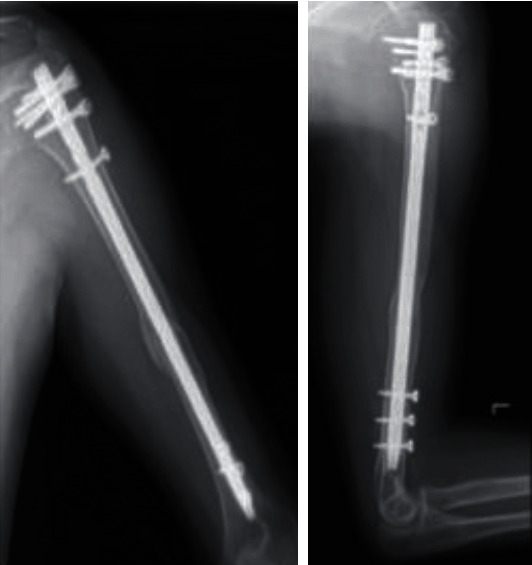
Radiographs at 7 months after the surgery. Bony union was obtained. At 15 months after the second surgery, we performed surgery to remove all implants including the screws and the nail.
